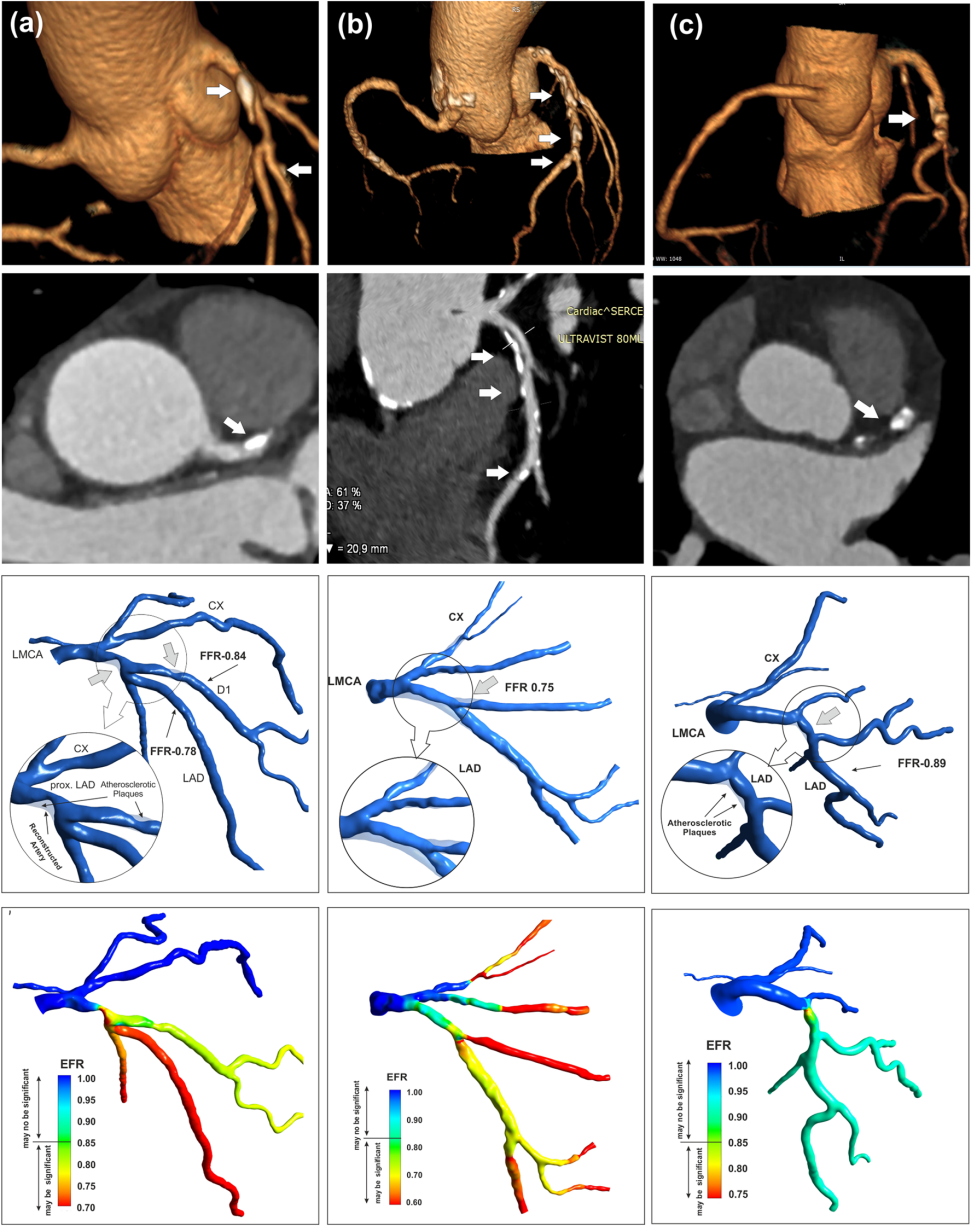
Example of EFR analysis of patient with different degrees of stenosis and different areas of their occurrence: (a) patient no. 2 with 60% narrowing and invasive FFR of 0.78 in proximal segment of LAD as well 50% narrowing and FFR of 0.84, measured in middle segment of diagonal branch D1, (b) of patient no. 2 with 60% narrowing and invasive FFR of 0.78 in proximal LAD as well 50% narrowing and invasive FFR of 0.84 in first diagonal branch, (c) of patient no. 22 with 60–70% narrowing and invasive FFR of 0.75 in proximal and middle LAD. From the top to bottom, respectively: volume rendering of LMCA, CT slice of normal and stenotic 3D models, EFR distribution in main branches.
